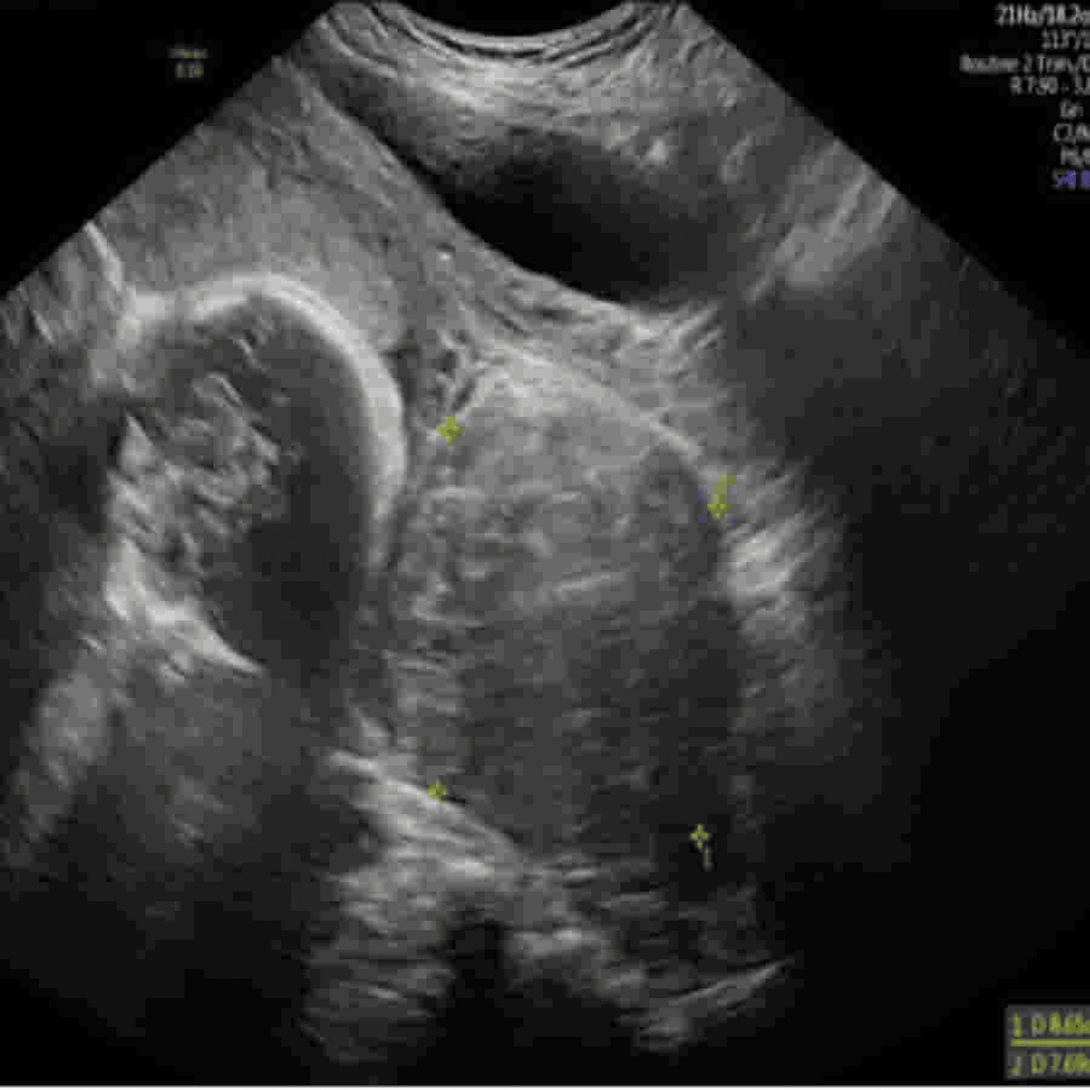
Transabdominal ultrasound showing a large hypoechoic uterine fibroid measuring approximately 8.65×7.69 cm, adjacent to the fetal head, contributing to uterine distortion during the second trimester.
